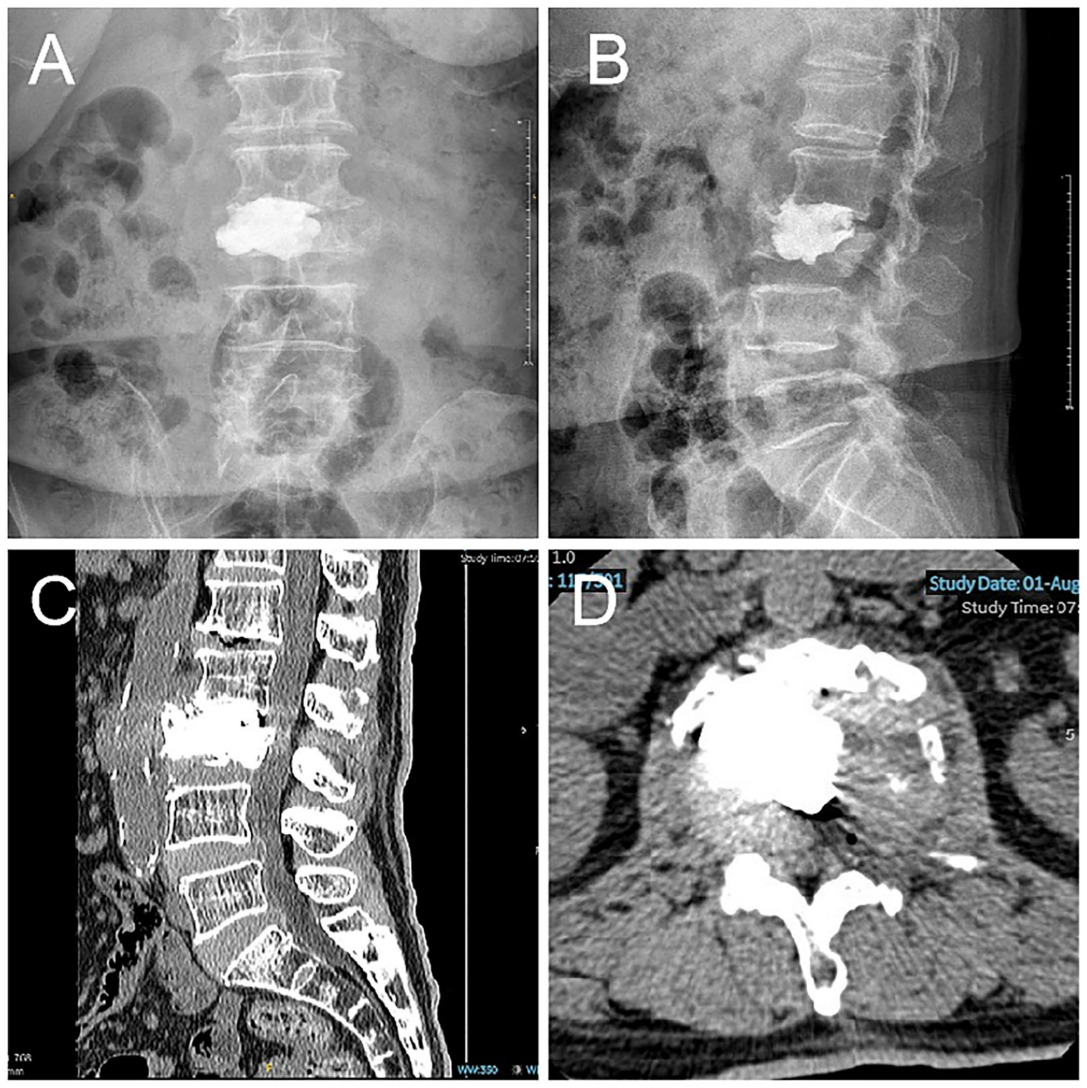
Postoperative lumbar CT scan indicates partial absence of intervertebral disk bone cement. (A–B) Lumbar Spine Digital X-Ray Radiography. (C–D) Lumbar spine CT.
